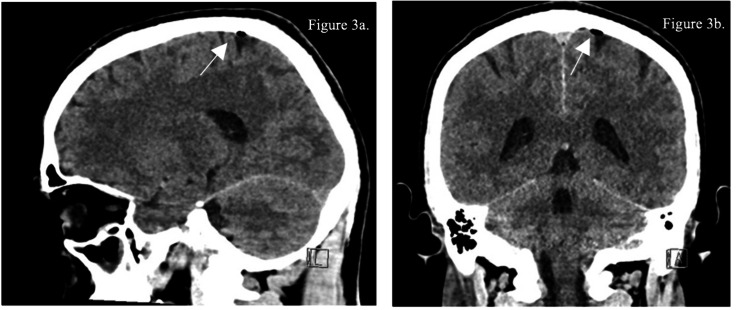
(a) Sagittal view of brain CT and (b) coronal view of brain CT showing air vacuole in the left frontoparietal region
